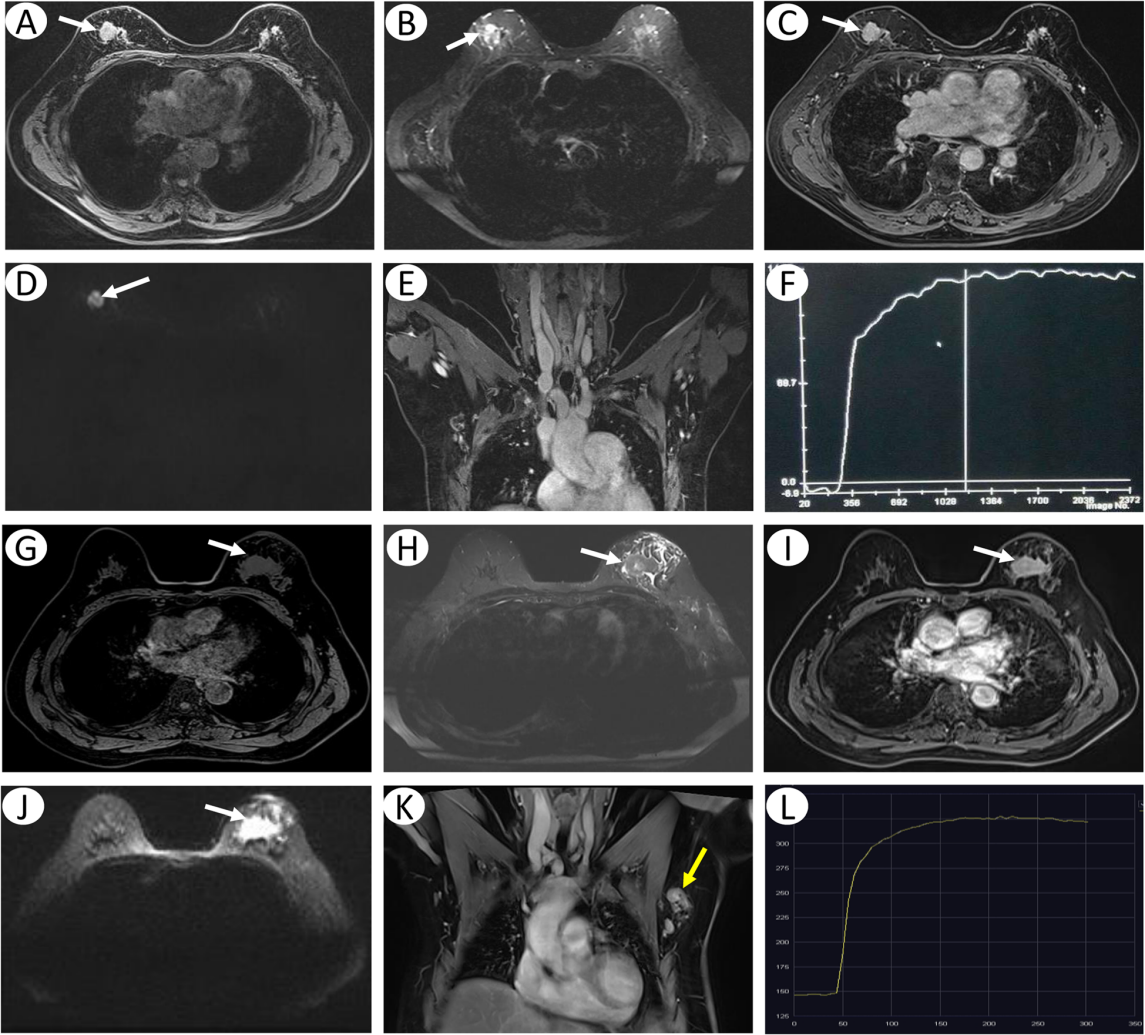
Examples of the scoring system in use. MRI examinations of patient 1 including. Axial T1-weighted fat-suppression (a), T2-weighted STIR (b), axial T1-weighted fat-suppression contrast-enhancement (c), DWI (d), TIC (e) and axillary imaging (f) showed a tumor (white arrows) with maximum diameter of 19 mm, location in the upper-outer quadrant, well-defined margins, absence of lobulation sign, obvious contrast-enhancement, marked hyperintensity on DWI images, type I TIC, and no ALNM. Biopsy results showed a IDC (grade 2) and positive HER-2 (+). The risk of LVI assessed by scoring system was 1. MRI examinations of patient 2 including axial T1-weighted fat-suppression (g), T2-weighted STIR (h), axial T1-weighted fat-suppression contrast-enhancement (i), DWI (j), TIC (k) and axillary imaging (l) showed a tumor (white arrows) with maximum diameter of 35 mm, location in the upper-inner quadrant, ill-defined margins, presence of lobulation sign, obvious contrast-enhancement, marked hyperintensity on DWI images, type II TIC, and multiple ALNM (yellow arrow). Biopsy results showed a IDC (grade 3) and positive HER-2 (3+). The risk score of LVI could be calculated to be 5 (Fig. 2n). Final pathology report showed this tumor had LVI
